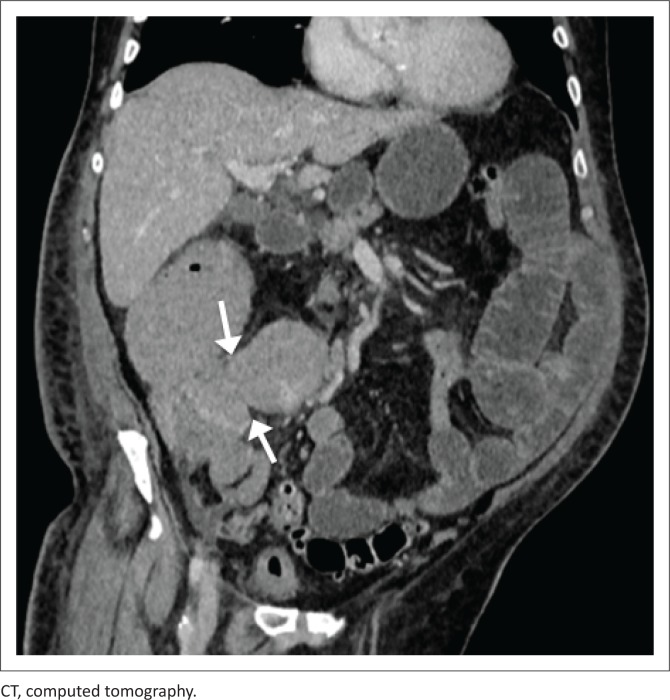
Coronal reconstruction of the portovenous phase of a CT abdomen of a 50-year-old male patient demonstrating lymphomatous circumferential thickening of terminal ileum and caecum (arrowed).
